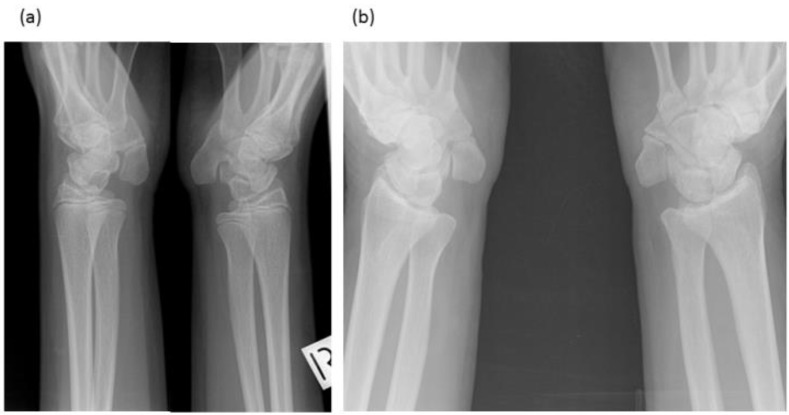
Radiographs of wrists of: (a) Proband—display bilateral symmetry of elongation of the pisiforms. (b) Proband’s father—displays bilateral symmetry of elongation of the pisiforms which are different in length and morphology from those of the proband. Both father and daughter had restricted wrist rotation/supination and grasping and writing capacity.
